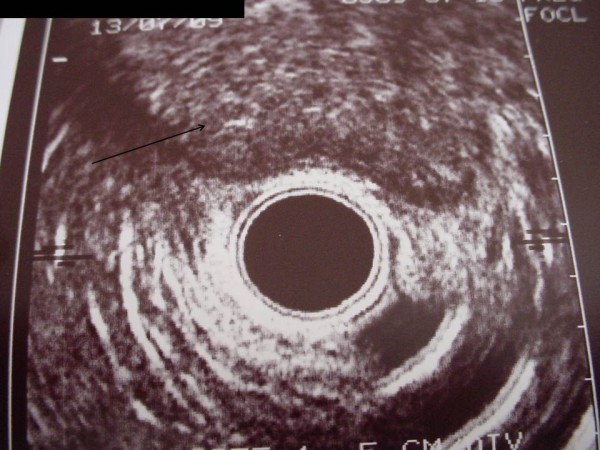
Endoanal ultrasound showing the perineal mass with sphincter involvement (black arrow).
